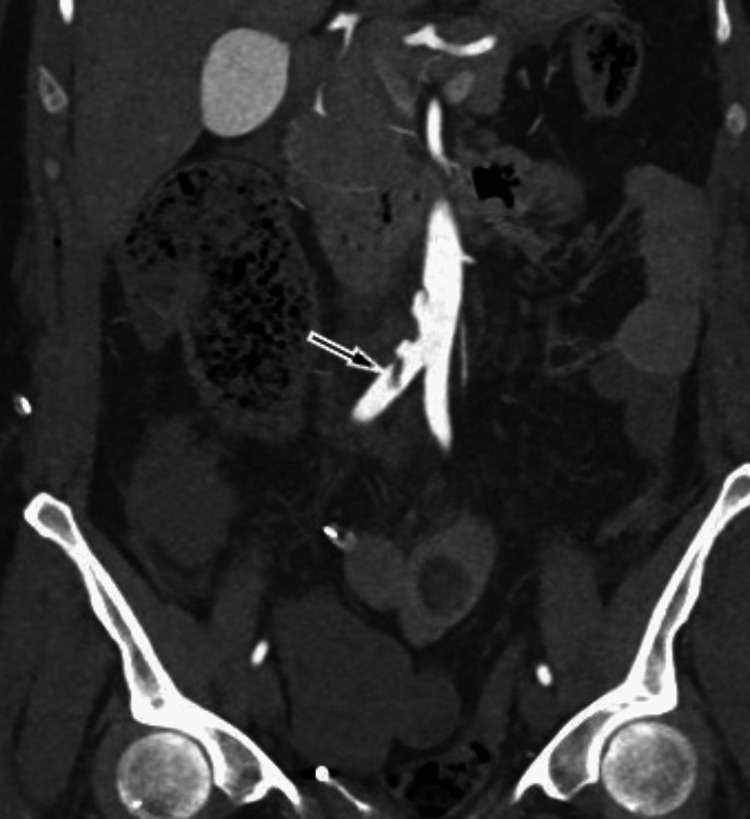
Control computed tomography angiography before EVAR, coronal image, slight progression of the thrombus in the right common iliac artery (arrow) EVAR: endovascular aortic repair.
